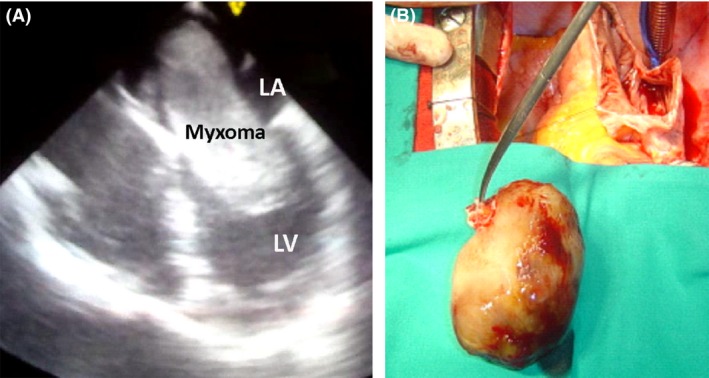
Echocardiography depicting left atrial myxoma causing left ventricular inflow tract obstruction (A). Intraoperative photo showing the excised myxoma (B).
